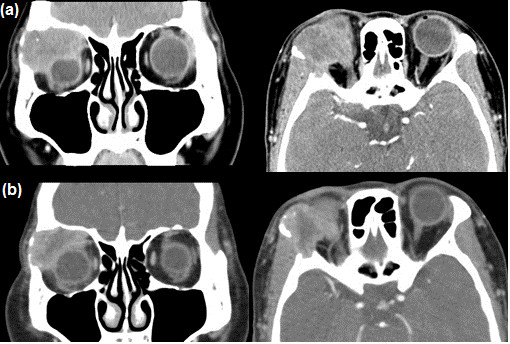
Patient 3 (45-year-old woman). (a): Computed tomography (CT) scan taken before neoadjuvant intra-arterial chemotherapy (NAIC) and (b) CT scan after NAIC. Note the reduction in tumour size.
